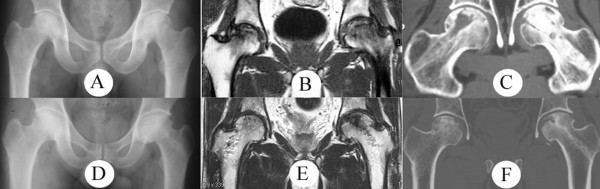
A) Preoperative AP radiograph of both hips shows round cystic change with a sclerotic rim and no femoral head flattening in either femoral head. B) Superior delineations of the necrotic areas in both femoral heads are seen on a T1-weighted, coronal, preoperative MRI image. C) Post-operative CT image of both femoral heads shows core decompression sites in both femoral heads and allograft impaction of the left femoral head. D) Both hip AP radiographs, E) MRI and F) CT images, were taken five years following surgery.
